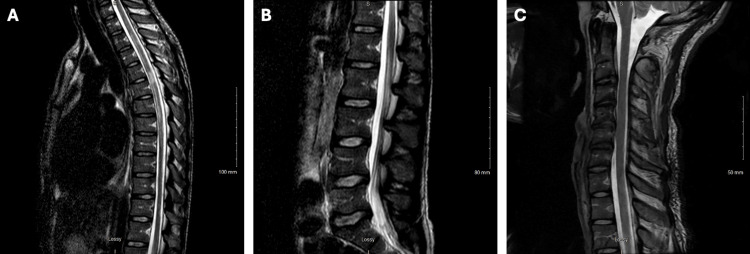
MR Imaging of Thoracic, Lumbar, and Cervical Spinal Cord in VZV Myelitis A: Sagittal T2-weighted sequence of the thoracic spinal cord with patchy hyperintense signal and mild enlargement of the cord, with the largest spinal cord hyperintensity at the T4-5 level involving most of the central cord. B: Sagittal T2-weighted sequence of the lumbar spinal cord with enhancement of cauda equina nerve roots and mild degenerative disc disease. C: Sagittal T2-weighted sequence of the cervical spinal cord with abnormal signaling visualized in the upper thoracic spinal cord extending superiorly into the cervical spinal cord at C7 level, no definite signal abnormality at C1-C6 levels, and mild degenerative disc disease. MR, magnetic resonance; VZV, varicella-zoster virus.
